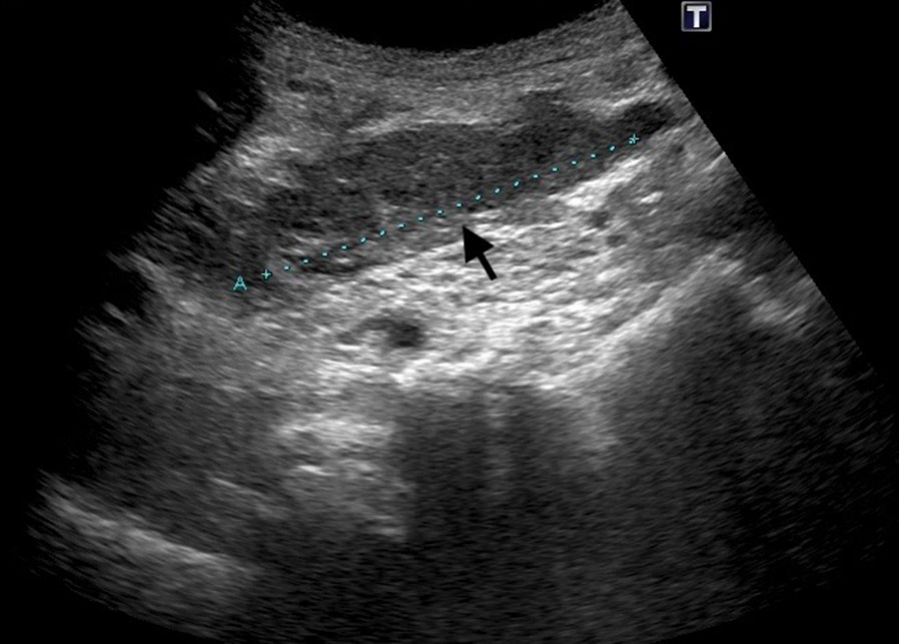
Abdominal ultrasonography. There was an echogenic region in the lumen of the blood vessel at a section less than 6 cm from the upper end of the superior mesenteric vein, which was considered a tumor thrombus (arrow)
